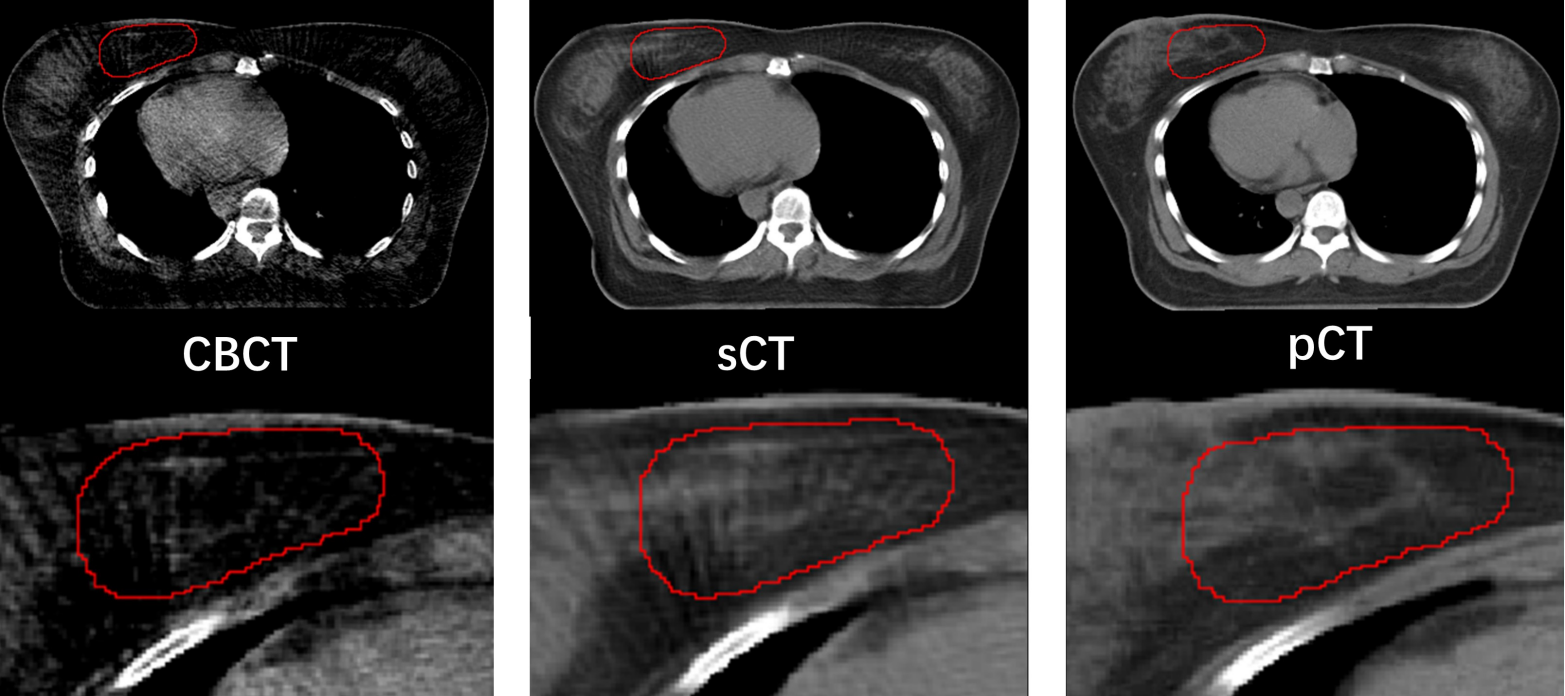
Comparison of soft tissue from CBCT, sCT, and pCT images with same window width and location, with tumor bed areas represented by the red contour line.
